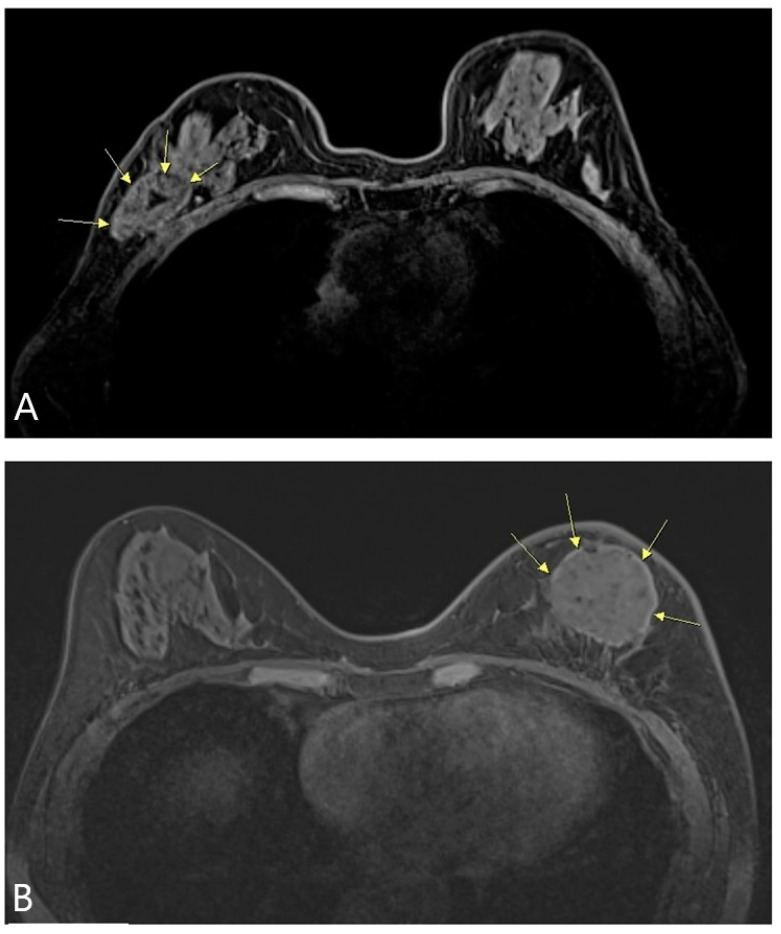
MRI on the breasts was performed after reconstruction using two types of ADM. (A) The sheet-type ADM (yellow arrows) was used in this BCS patient. (B) The pellet-type ADM (yellow arrows) was applied for reconstruction after BCS. ADM, acellular dermal matrix; BCS, breast-conserving surgery; MRI, magnetic resonance imaging.
